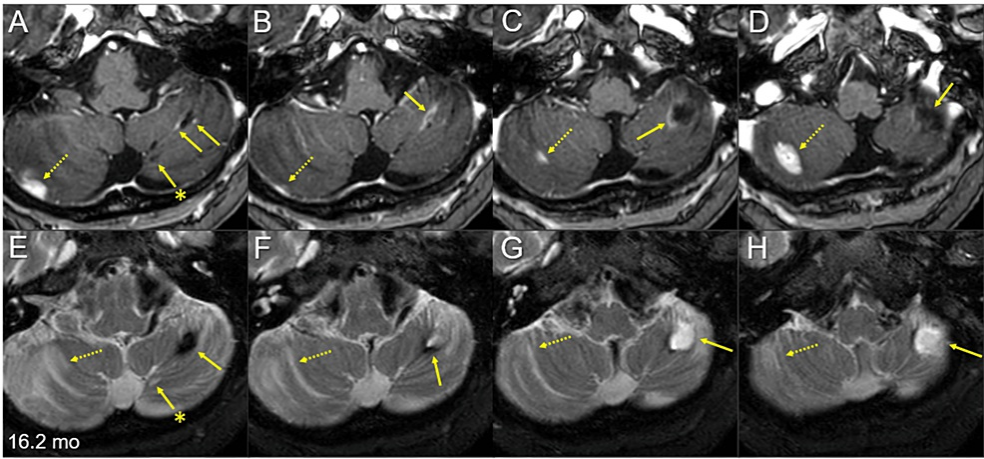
Multiple magnetic resonance images of the posterior fossa for the target definition of salvage stereotactic radiosurgery 16.2 months after whole-brain radiotherapy The images show axial CE-T1-WIs (A-D) and axial T2-WIs (E-H). (A-H) These images are shown at the same magnification and coordinates under co-registration and fusions. Alphabetically from cranial to caudal (A-D, E-H). The large left cerebellar lesion regressed remarkably, leaving the cavitary remnant (arrows in A-H), in which the periphery was partially enhanced (arrows in A-C) and partially hypointense on T2-WIs (arrows in E, F). The adjacent medial lesion regressed completely, only leaving the tiny cavitary scar (arrows with asterisks in A and E). Two discontinuous solid-enhancing lesions (dashed arrows in A-D) developed in the right cerebellar hemisphere, which is associated with perilesional edema (dashed arrows in E-H). mo: months; CE: contrast-enhanced; WIs: weighted images
